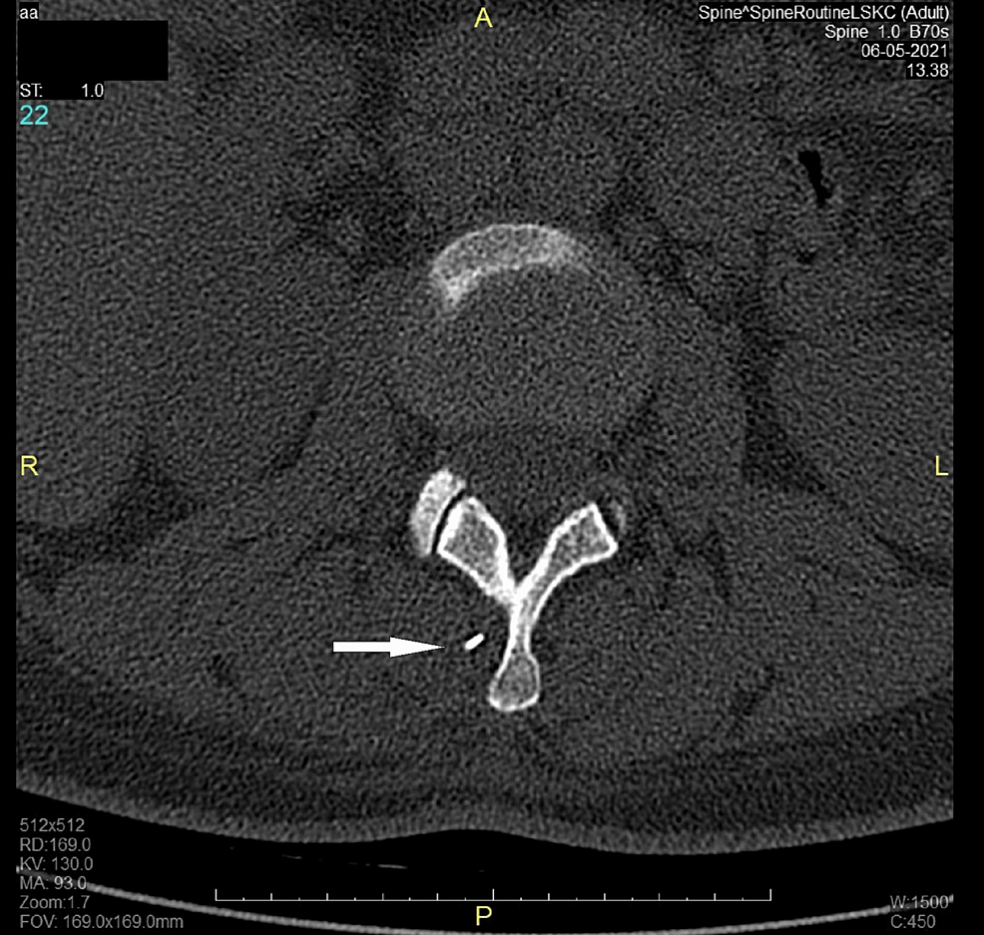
CT scan of the lumbar spine - image 2 The horizontal plane image shows the tip of the needle (white arrow pointing toward the tip of the needle) close to the lateral side of the L2 spinous process, within the right paravertebral space of the erector spinae muscle CT: computed tomography
